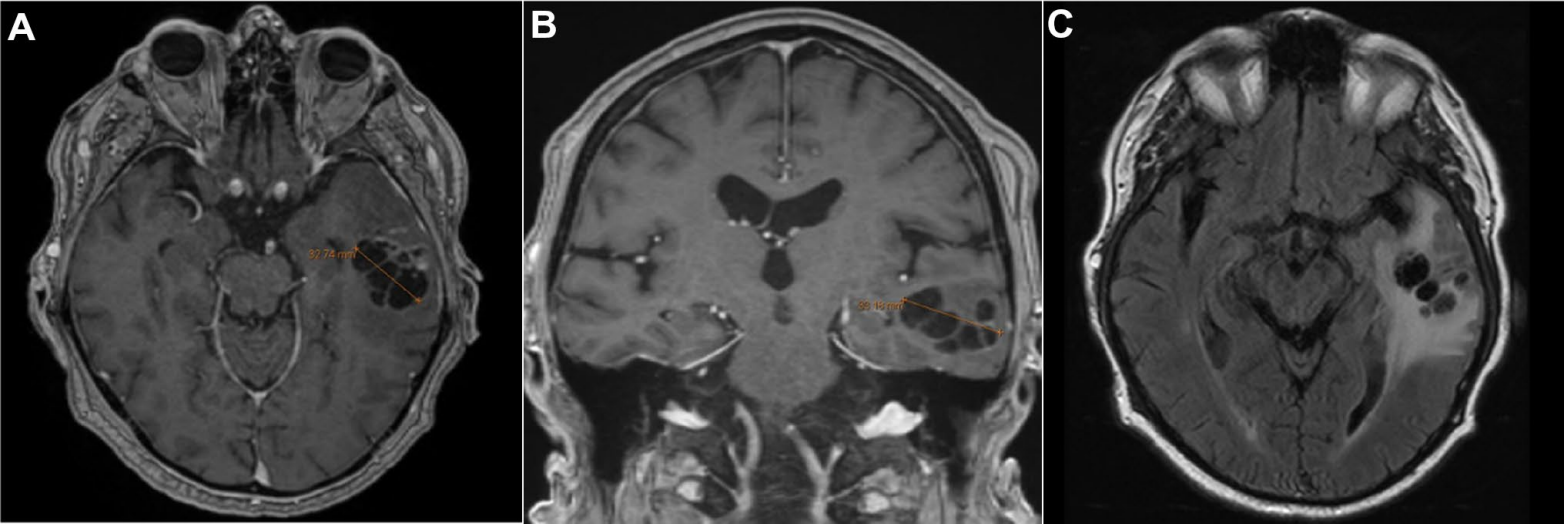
Magnetic resonance imaging (MRI) of an intracerebral, polycystic lesion of the left temporal lobe. The transverse view (A, T1-weighted image, contrast-enhanced) and the coronal view (B, T1-weighted image) show the lesion of approximately 30 × 30 mm in size in the left temporal lobe. The transverse view (C, Turbo Inversion Recovery Magnitude (TIRM) imaging) shows the perifocal edema caused by the lesion which was suspected to be a malignoma. No other intracerebral cysts could be detected. A slight midline shift can be observed. All images were acquired pre-surgery
